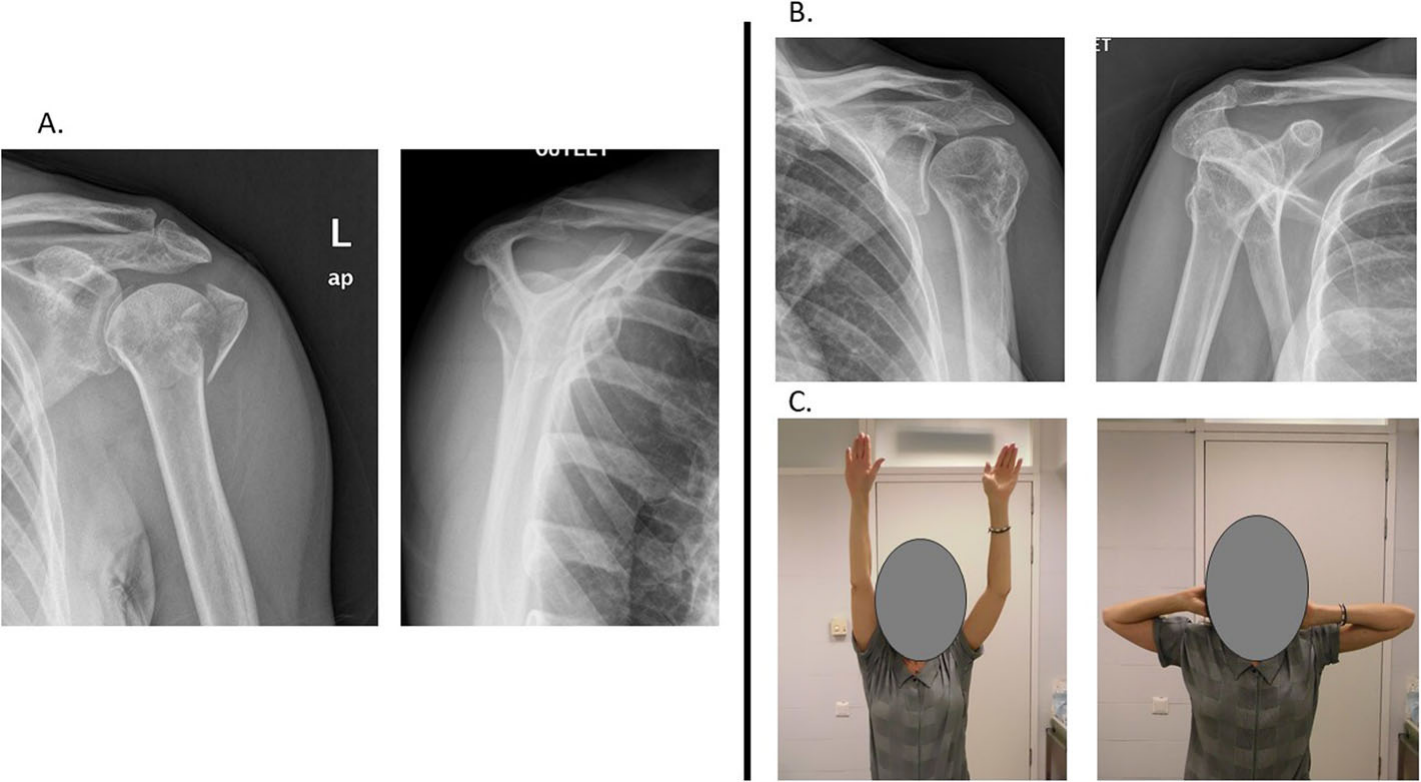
A Initial X-ray presentation of a 47-year-old. Female with a proximal humeral fracture. B Radiological results achieved at the 2-year follow-up. C Clinical results at the 2-year follow-up with a Constant-Murley score of 75 points
